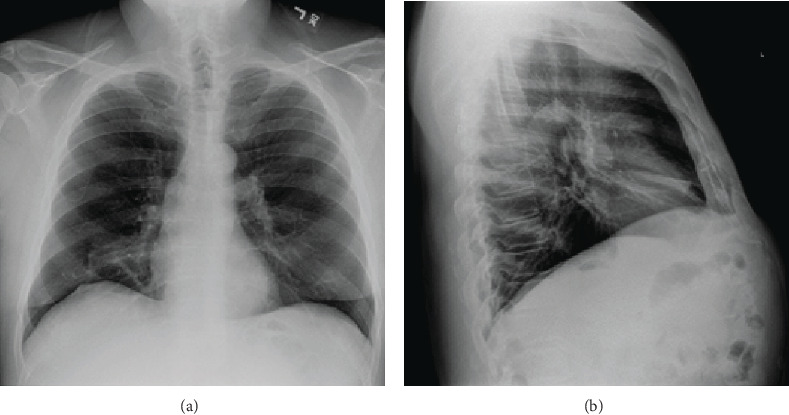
Poster anterior and lateral chest X-ray: right middle lobe infiltrate, band-like in shape, overlying the anterior cardiac contour.
